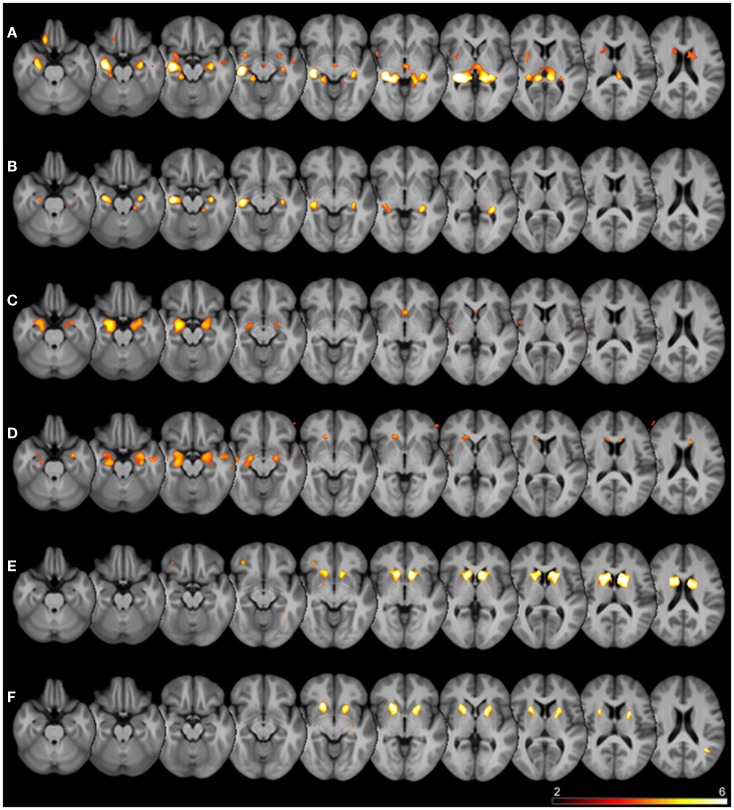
Superimposed SPM results. Thresholded SPM results (uncorrected *p* < 0.0001) superimposed on the averaged T1-weighted images of all subjects are shown. The color scale represents SPM t-scores, images are in radiological convention (left in image is right in the subject). Rows are different seed regions: (A) left hippocampus, (B) right hippocampus, (C) left amygdala, (D) right amygdala, (E) left caudate nucleus, (F) left putamen.
